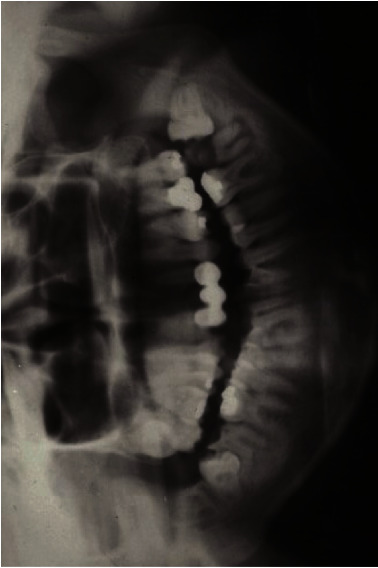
Panoramic radiograph showed that horizontal impaction of #17 was noted. The apical part of #17 was fully formed.
